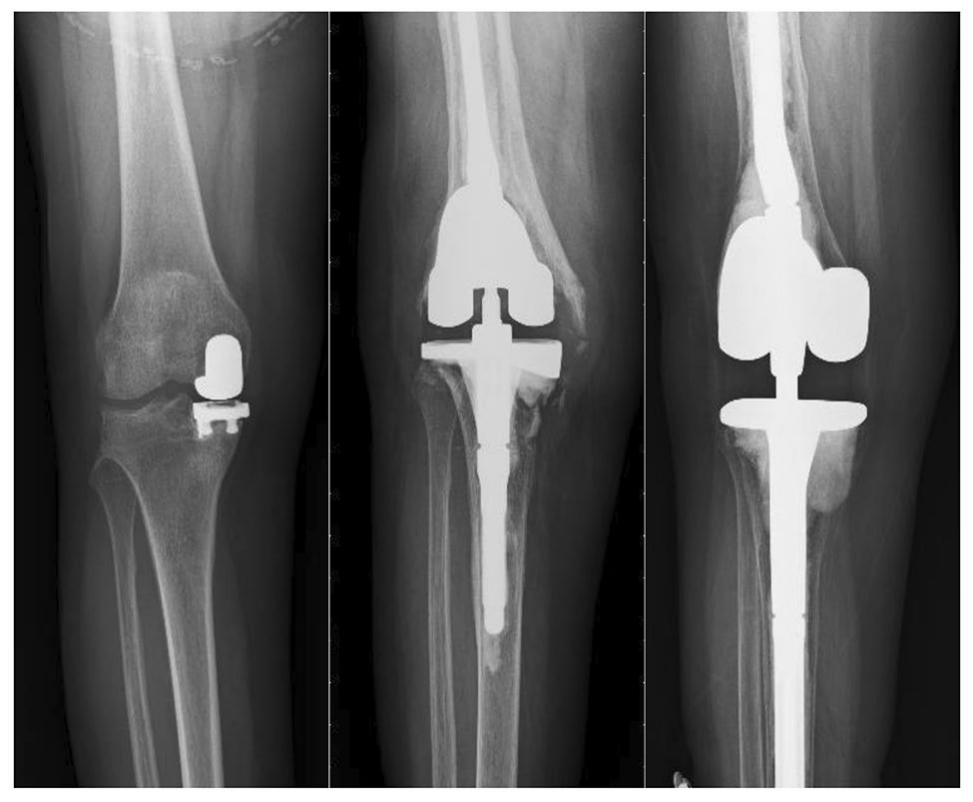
Anteroposterior radiograph of Patient B’s knee. Unicompartmental knee arthropasty (UKA) was performed in the past (left), procedure with revision prostheses because of bone defect after UKA failure (center), and procedure with revision prostheses after the failure of the original prosthesis because of periprosthetic joint infection (right)
